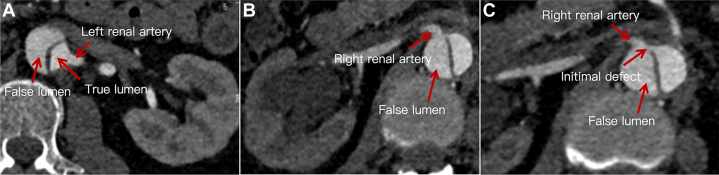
High-Resolution Computed Tomography Angiography Axial Views of Renal Artery Involvement (A) The left renal artery originates exclusively from the true lumen. (B and C) The right renal artery exhibits a complex origin; although its orifice is involved by the false lumen, an intimal defect (arrows) provides a patent perfusion channel from the true lumen. This anatomical identification (Nagamine subtype III-a) is the decisive factor for the feasibility of renal denervation access.
